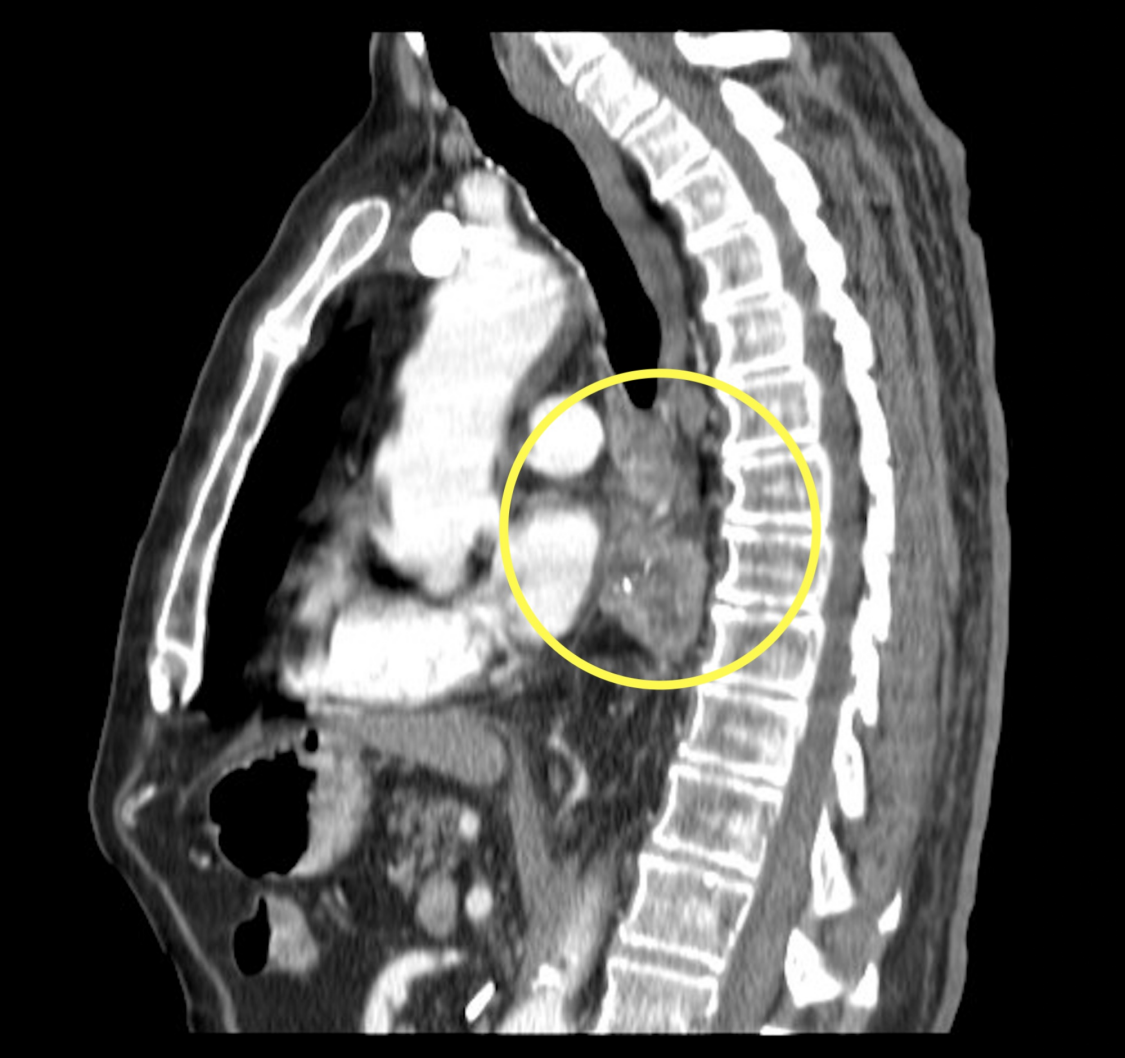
Sagittal contrast enhanced computed tomography (CT) chest (soft tissue window) demonstrating a solid heterogeneously enhancing esophageal mass (yellow circle).
